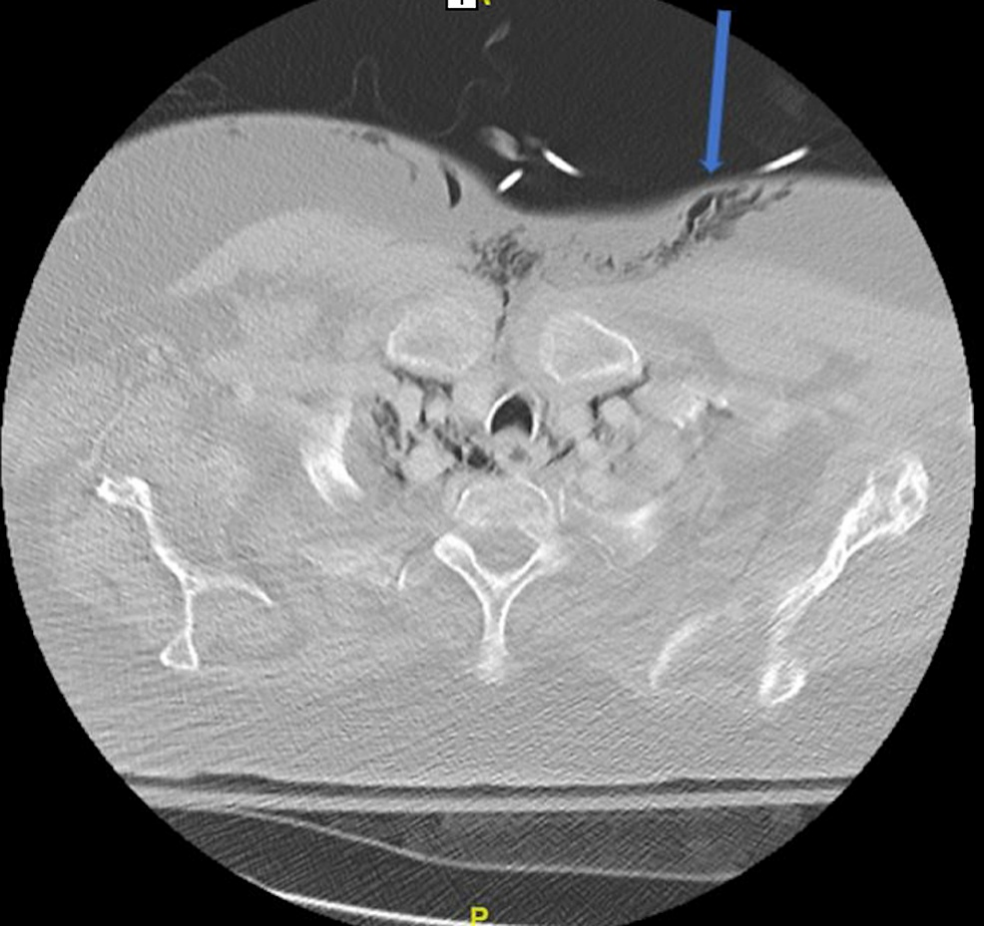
Computed tomography chest showing subcutaneous emphysema (blue arrow)
